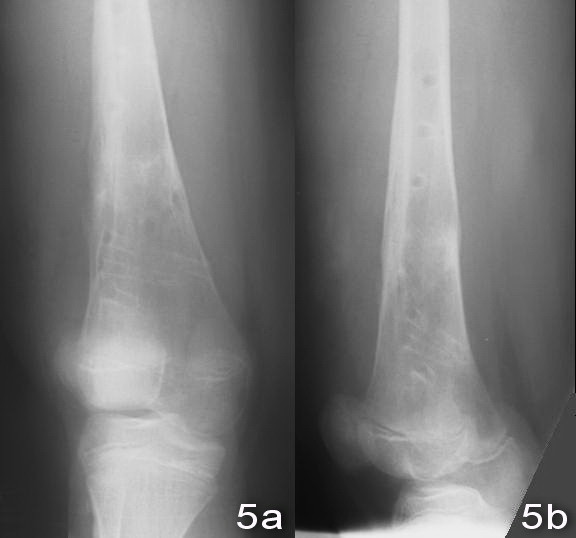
X-ray of right knee after implant removal.
